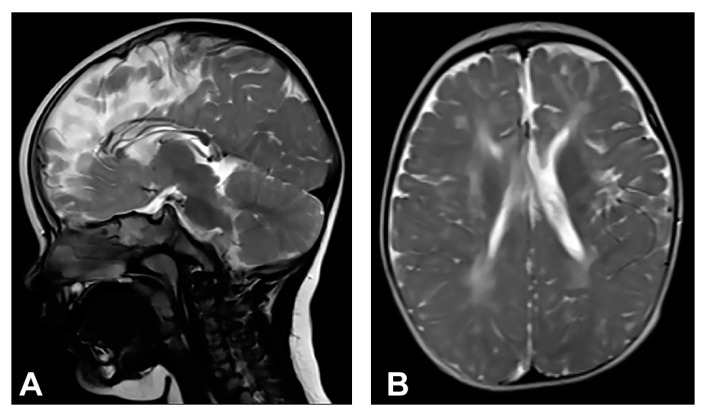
(Case 2) (A) Sagittal T2W magnetic resonance image at the age of one shows CC hypoplasia especially of the rostrum and body. (B) Axial T2W magnetic resonance image shows brain atrophy, supratentorial white matter reduction with elevated IS on T2W/FLAIR and gliotic changes. Mild bilateral non-progressive ventriculomegaly is more prominent on the left.
